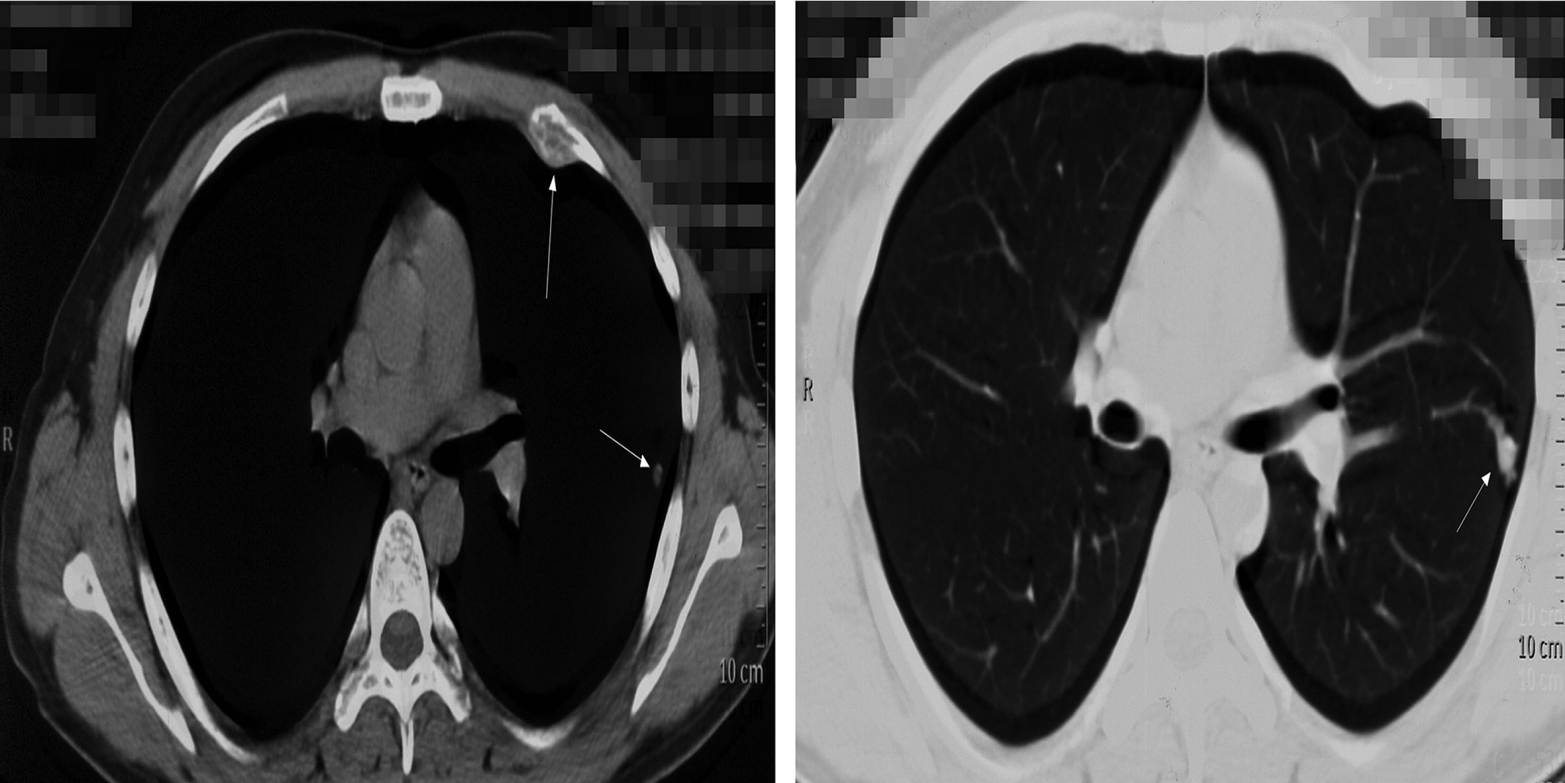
Thoracic CT demonstrated the metastatic lesions of the rib, left lung lobe. White arrows indicate the lesions.
